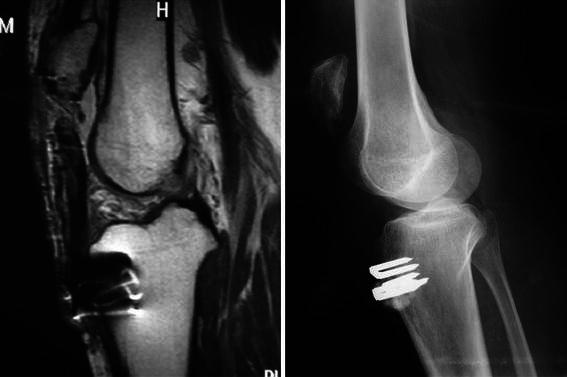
X-ray and MRI images of the torn patellar tendon after the two previous operations
